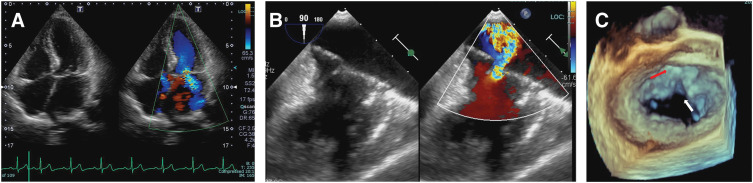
Preoperative echocardiography showing (A) the ostium primum defect and the absence of the ventricular septal defect, (B) left atrioventricular valve regurgitation. (C) 3D image showing the cleft of the left atrioventricular valve (white arrow) and atrial septal defect (red arrow).
